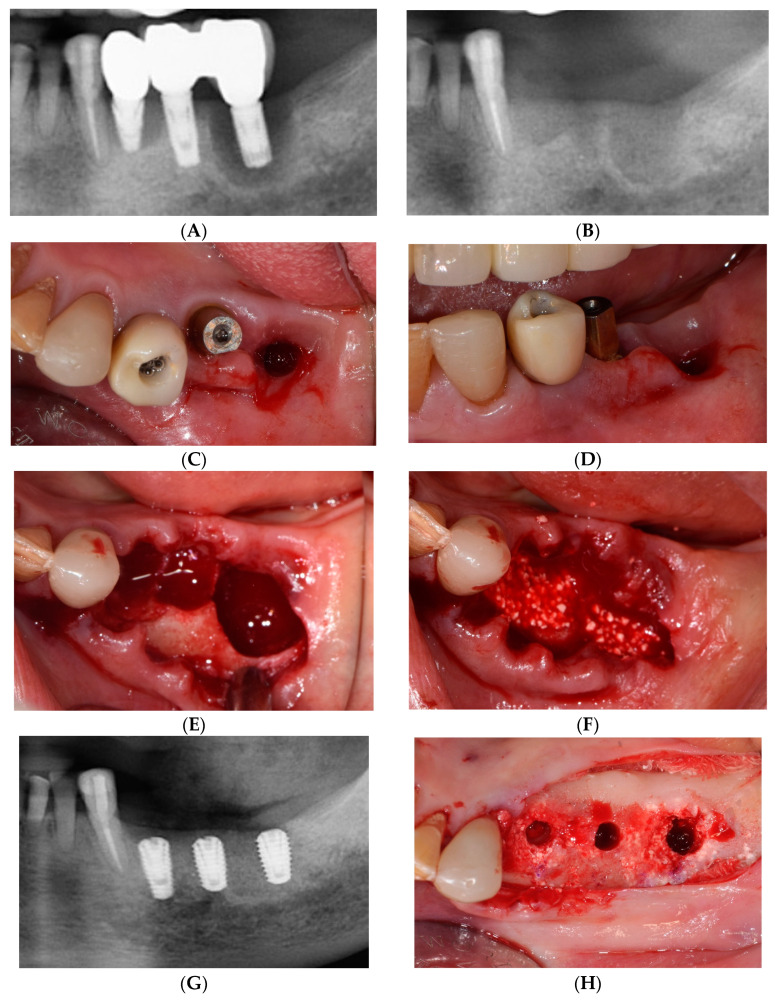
(A) Panoramic radiograph at the initial examination. (B) Panoramic radiograph after alveolar ridge preservation (ARP). (C) Occlusal and (D) buccal view at the initial examination. (E) Fixture removals. (F) Bone grafting for ARP using an octacalcium phosphate-based synthetic bone substitute. (G) Panoramic radiograph 4 months after ARP. (H) Implant placements.
